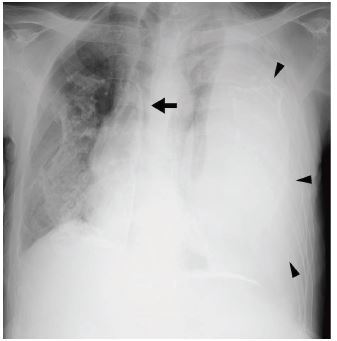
Chest radiograph (CXR) on the patient’s arrival to the emergency department. The CXR (anterior-posterior) before treatment shows bilateral, asbestos-calcified pleural plaques, a completely opaque left thorax (black arrowheads), and mediastinal shift toward the right (black arrow).
